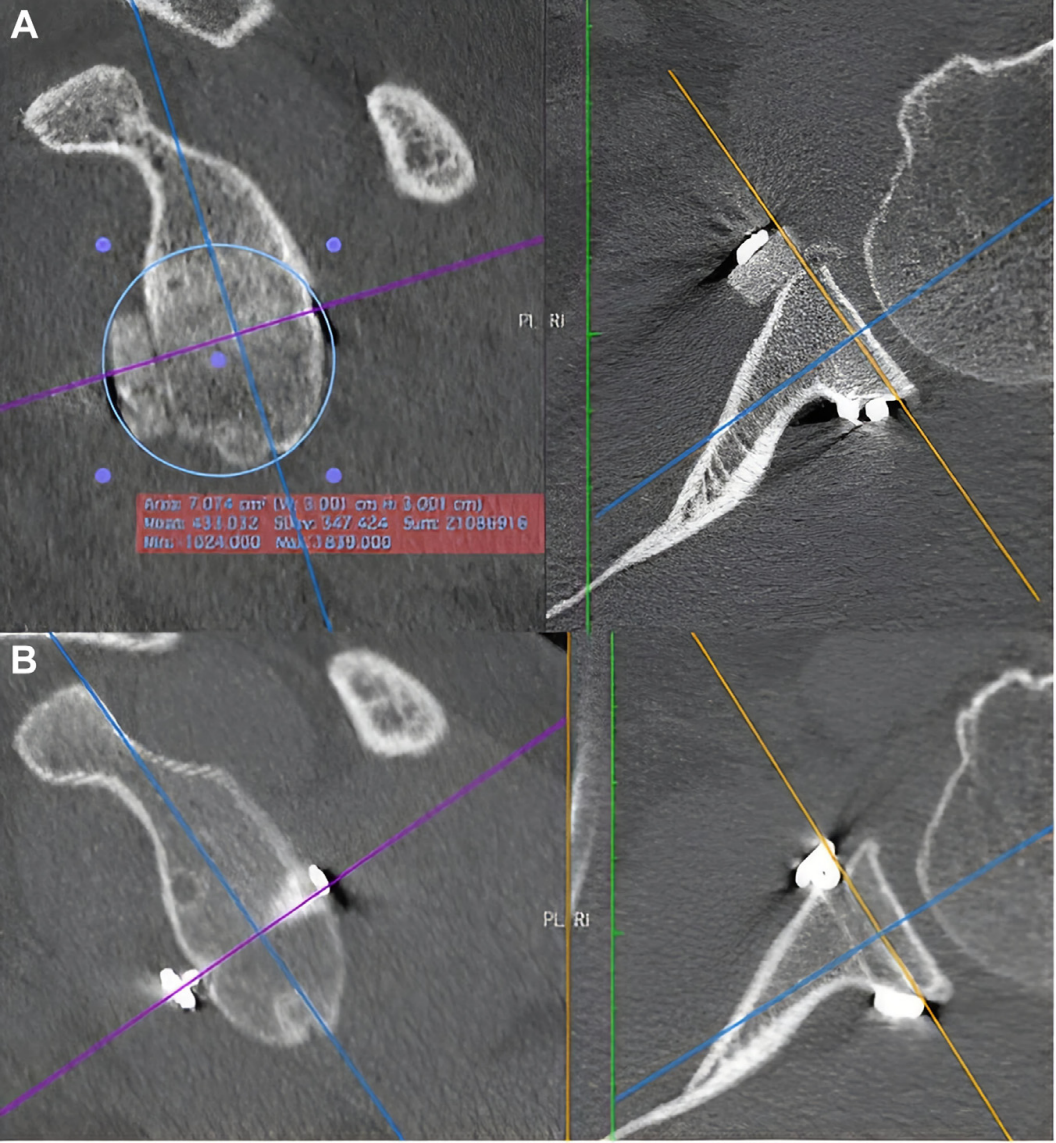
(A) 15 days postoperative and (B) last follow-up CT scan evaluation, showing bone graft complete resorption. *CT*, computed tomography.
